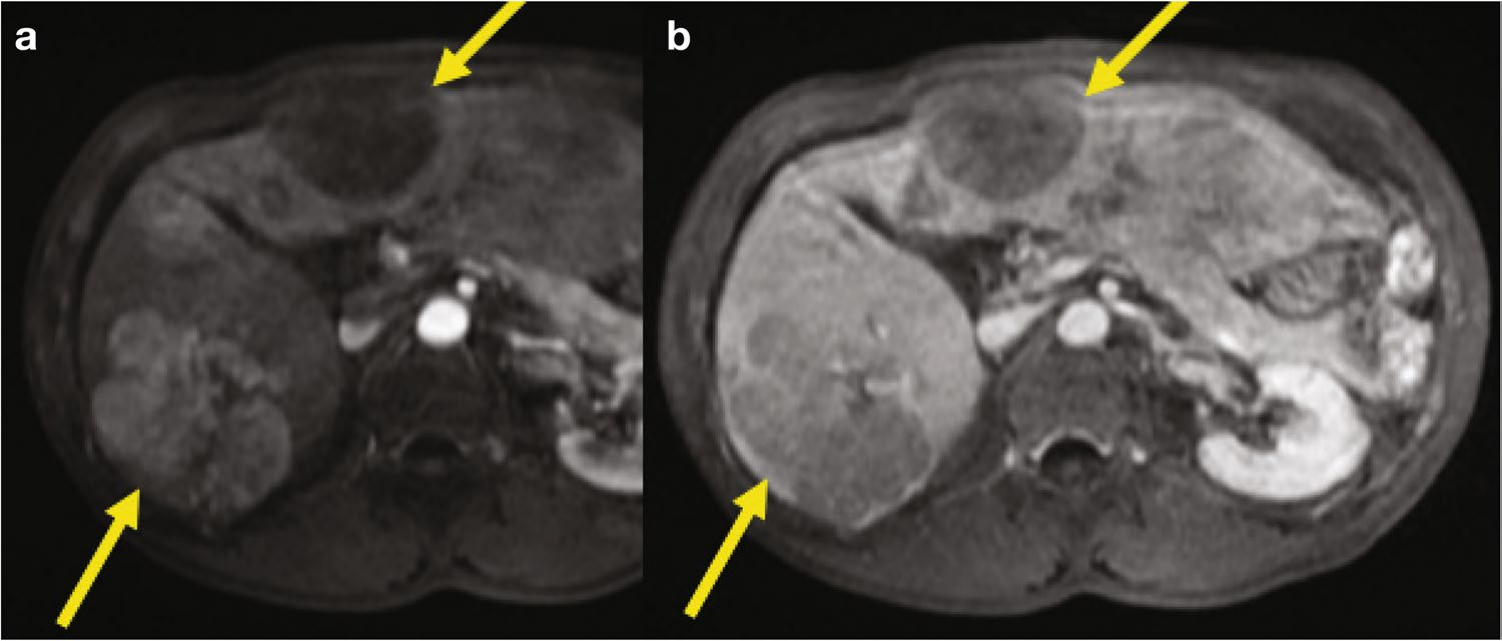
Sixty-nine-year-old male with HCV cirrhosis and HCC. Gadoxetic acid-enhanced T1-weighted images during the arterial (a) and PVP (b) demonstrate two large infiltrative tumors: an arterially hyperenhancing tumor in the right hepatic lobe (right arrow) and a venous-enhancing tumor in the left hepatic lobe (left arrow). *Abbreviation: PVP,* portal venous phase
